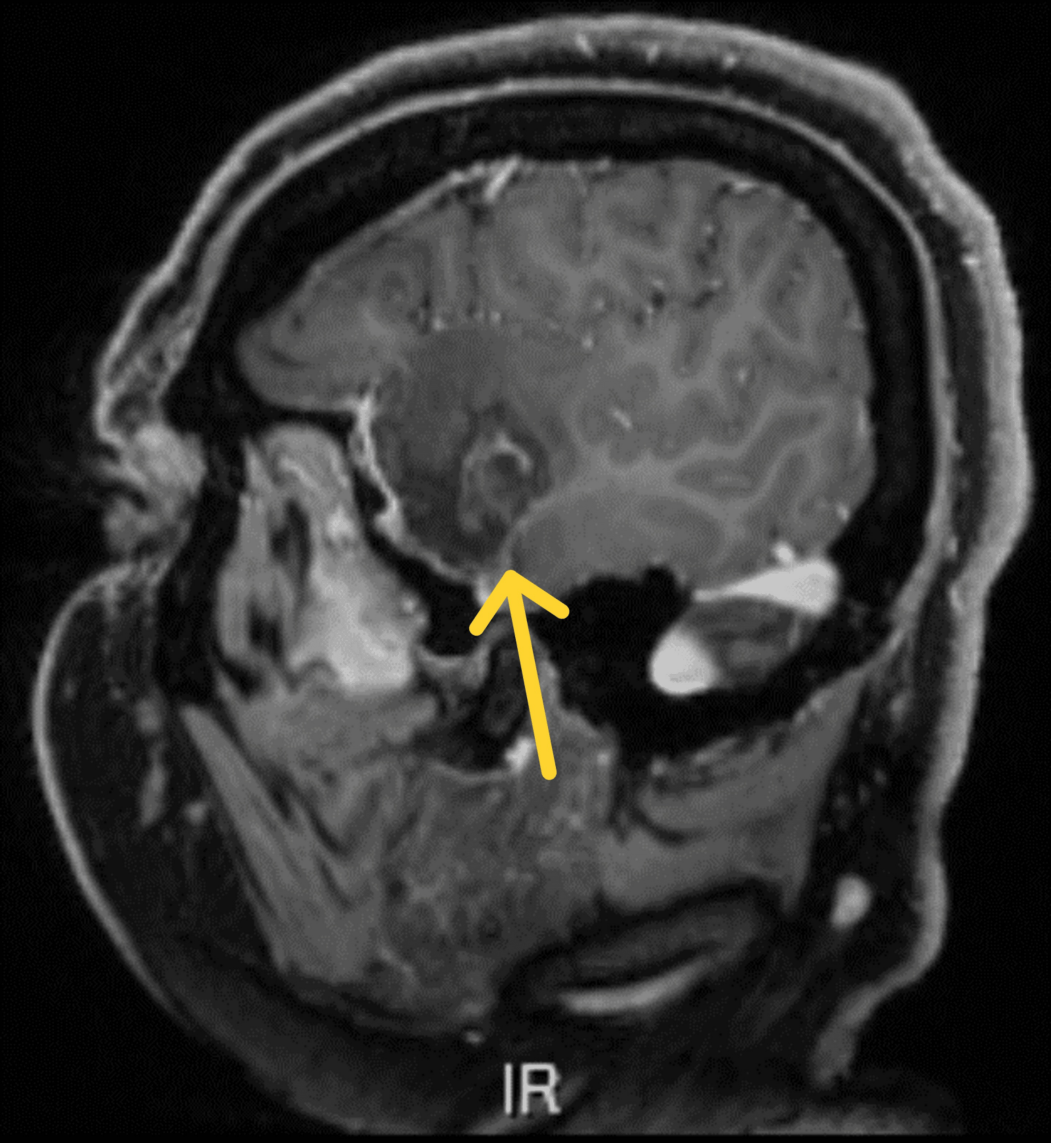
Follow-up MRI approximately 12 months postoperatively (T1-weighted with contrast, sagittal view) showing tumor recurrence in the right temporal lobe.
